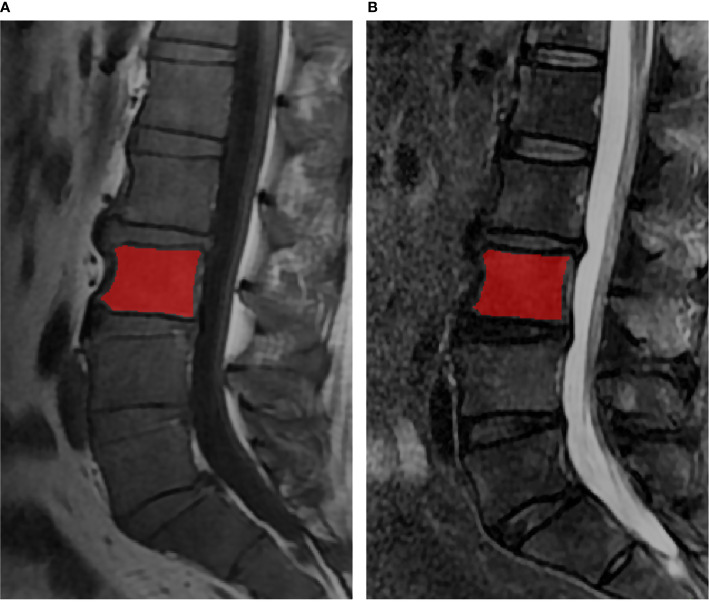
The lumbar MRI examination for multiple myeloma. The red regions were representative ROI segmentations of the vertebral bodies in T1WI (A) and T2WI (B).
